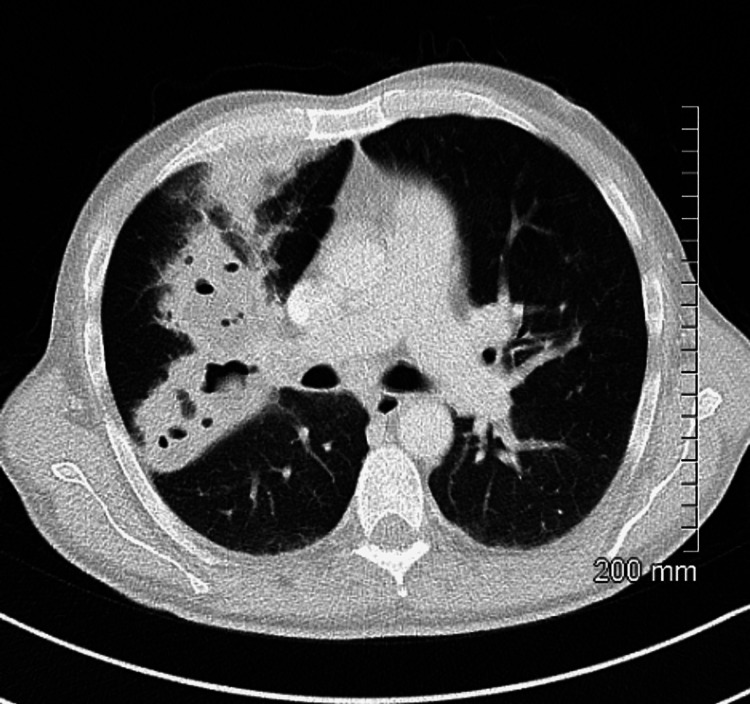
CT chest on admission showing the confluent intrapulmonary lesion suggestive of lung abscesses
